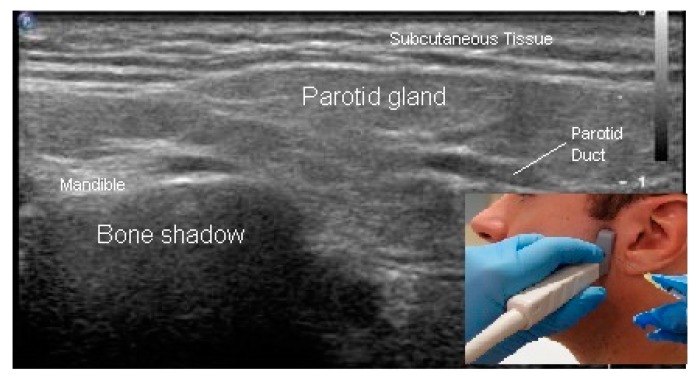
B-mode ultrasound image, parotid salivary gland.
